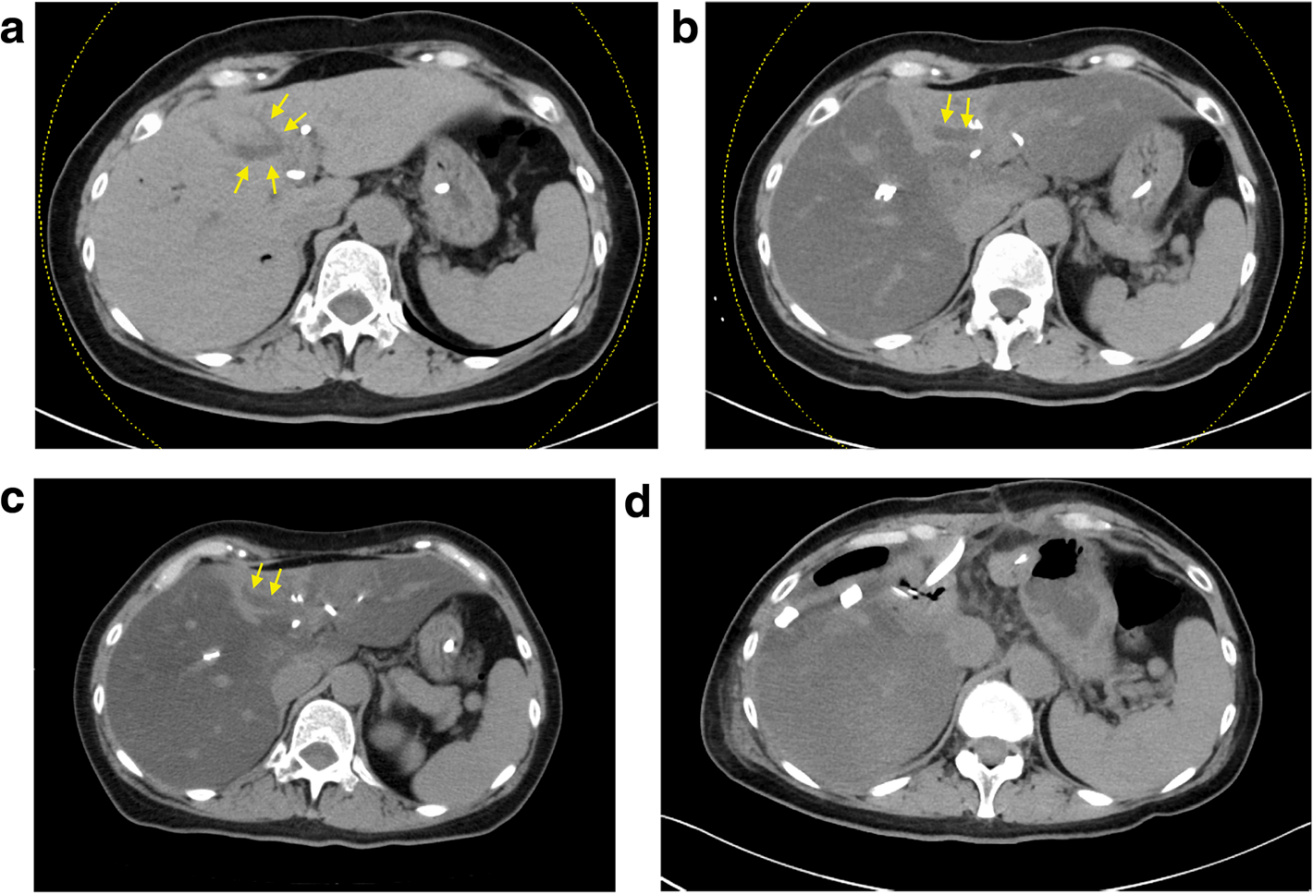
CT of the liver at different stage. a CT of the liver before PVE. Yellow arrows, undrained (dilated) bile ducts (b) CT after PVE demonstrating acute fatty change of the liver and sparing of segment IV. Yellow arrows, undrained (dilated) bile ducts (c) CT 6 weeks after PVE (d) CT of the liver remnant 1 week after trisectionectomy
